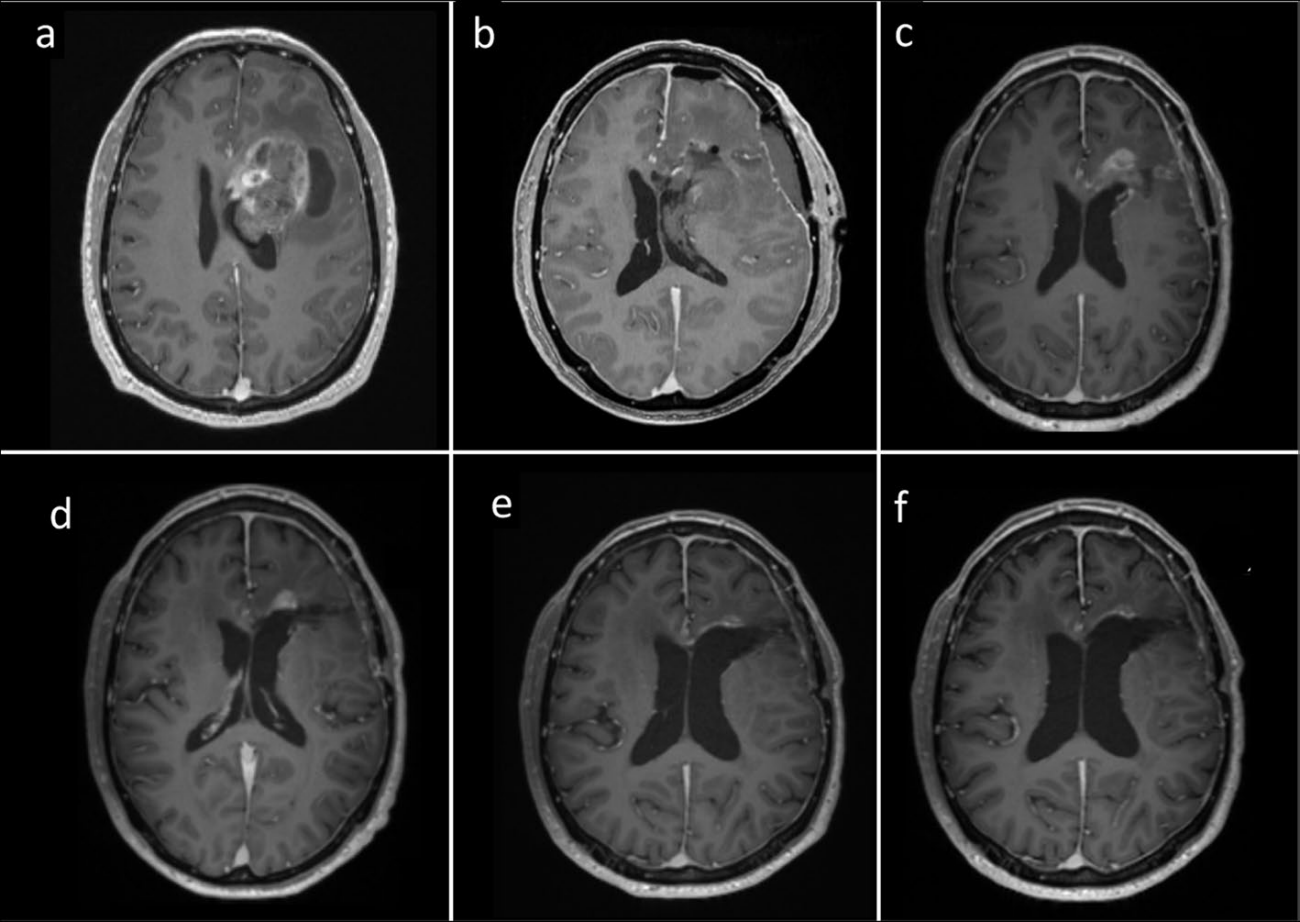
Contrast enhanced MRI studies of one patient (#5) a before surgery, b postoperatively, c after completed Salovum therapy, d at 12-month follow-up, e at 18-month follow-up and f at 24-month follow-up. This patient displayed increased contrast enhancement after the concomitant therapy followed by diminishing enhancement and durable tumor control, possibly due to initial radionecrosis followed by delayed immunologic response to therapy
